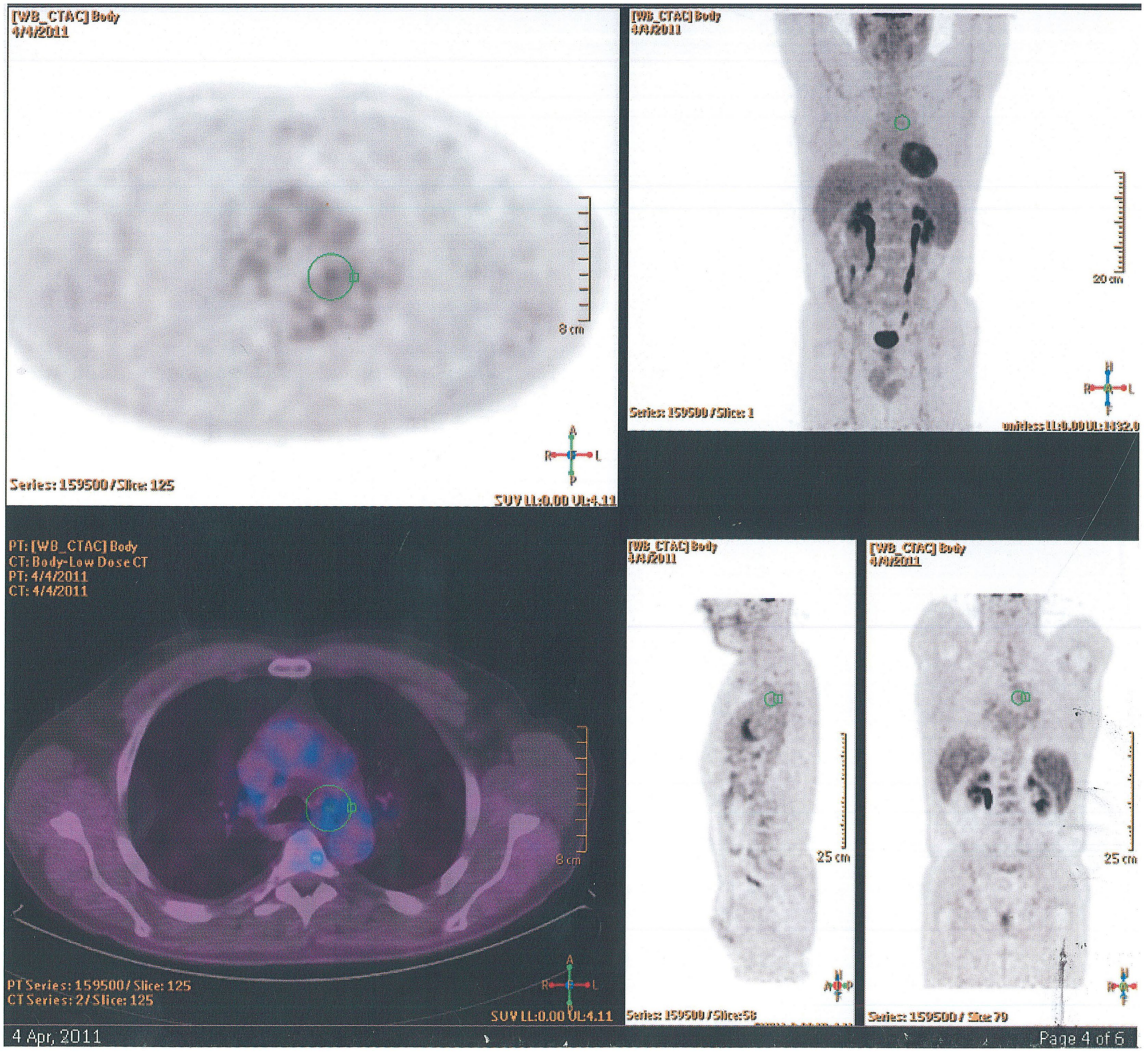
^18^F-FDG PET/CT findings in eosinophilic granulomatosis with polyangiitis. Axial, coronal, and sagittal images show paracardial nodular granulomatous infiltrate.
